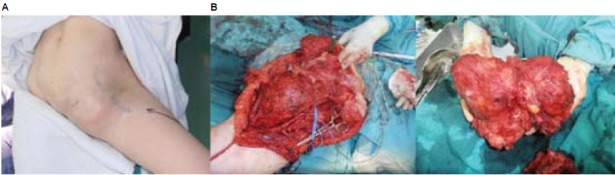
Fig. 2: Plain radiographs of Pelvis – Anterior posterior (AP) views (right) and Lateral views (left) F2:
